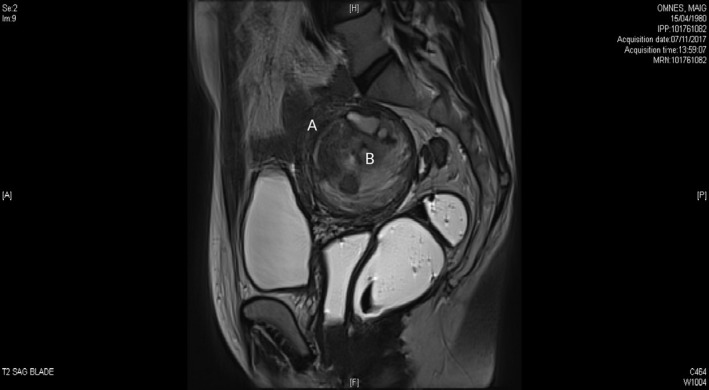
Sagittal cut in MRI—T2‐weighted sequence: The uterine mass (B) is heterogeneous, distorting the uterine cavity visible in the form of a thin‐edged in antero‐superior (A)
